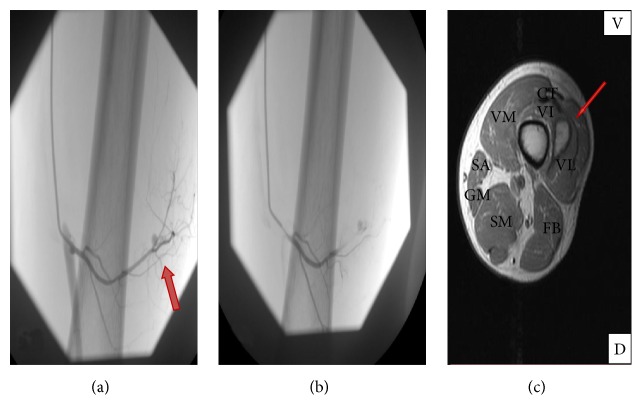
(a) A selective arteriography shows a point of active bleeding in a branch of the femoral artery at the level of the femoropopliteal junction (red arrow). (b) The image shows selective catheterisation of the bleeding branch (using Spongostan® particles) to carry out the correct occlusion of the branch. (c) Nuclear magnetic resonance (axial thigh T1) is performed after a month to monitor the patient's evolution. Subacute haematoma is observed in the distal third of the left thigh in the anterolateral compartment within the vastus intermedius muscle (VI). The haematoma did not present signs of spreading to other compartments or of affecting the adjacent femur. V: ventral. D: dorsal. VM: vastus medialis. VL: vastus lateralis. CT: quadriceps tendon. SA: sartorius. GM: gracilis muscle. FB: femoral biceps. SM: semimembranosus and semitendinosus muscle. Red arrow: subacute haematoma in resolution.
